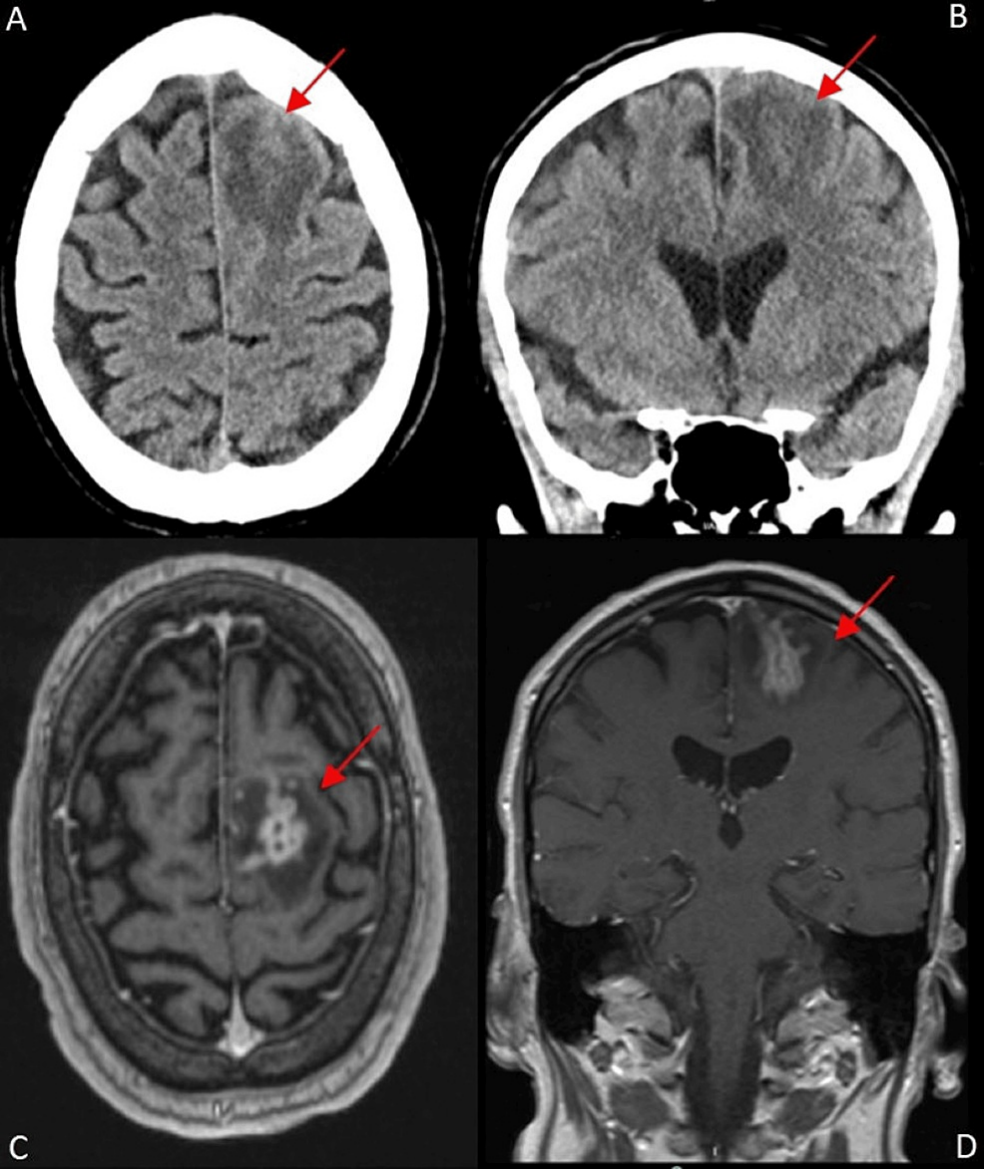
Axial and coronal CT head and MRI revealing left intra-axial frontal lobe lesion with edema Panel A: axial CT head without contrast, panel B: coronal CT head without contrast, revealing a left intra-axial frontal lobe lesion measuring 1.7 x 1.3 cm with surrounding edema (arrow), panel C: axial MRI brain with contrast, and panel D: coronal cuts revealing an intra-axial peripherally enhancing lesion centered in the medial posterior left frontal lobe surrounded by vasogenic edema with some areas of tubular appearance measuring 1.6 x 1.9 x 2.2 cm (arrow) with minimal mass effect and no midline shift.
